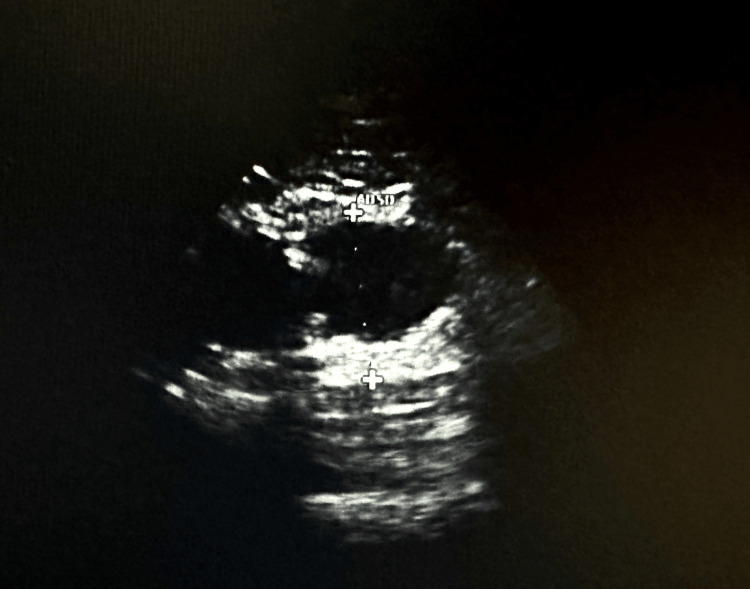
Abdominal ultrasound demonstrating the infrarenal aortic aneurysm measuring 4.4 cm in the distal aorta.
